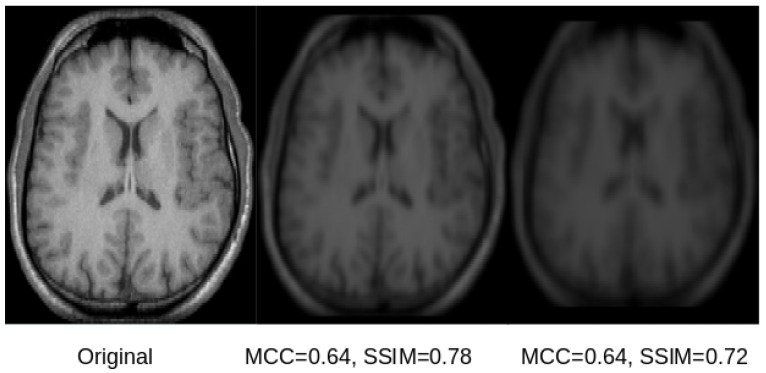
A selected slice from an original MRI dataset without skull stripping (left) and its reconstruction from the latent space of the U-Net (center) and SegNet (right). As can be observed in the image, the original and the reconstructed images are overall similar. However, the reconstruction from the SegNet model is more blurry, which is reflected by lower a SSIM score, while the MCC is very similar for both reconstructions, not reflecting the differences in reconstruction quality.
